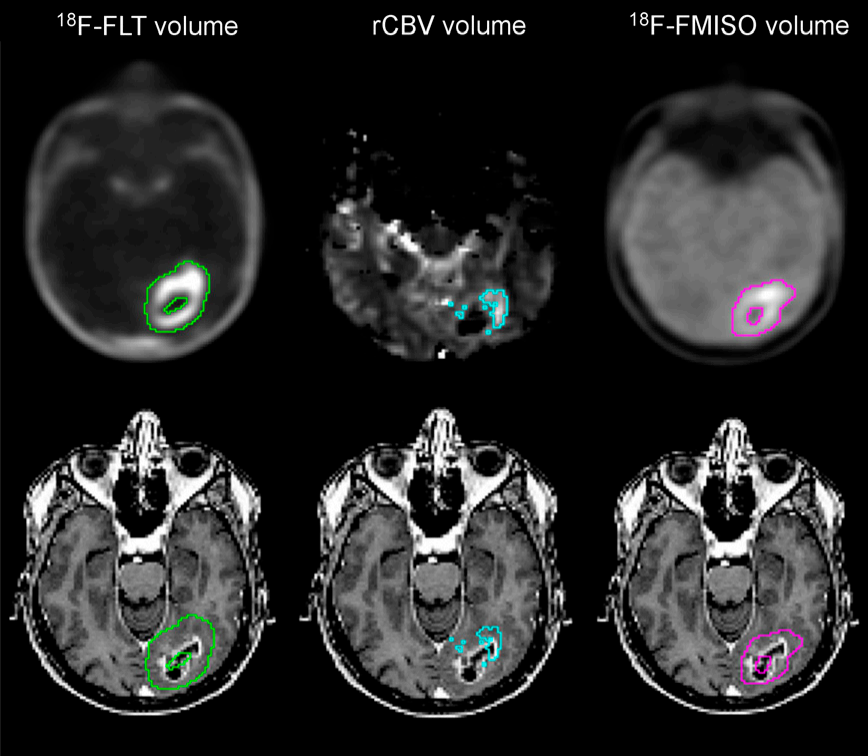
Example of proliferative volume in green, hypervascularized volume in blue, and hypoxic volume in pink on segmented (top) and overlaid (bottom) 3D T1w-Gd MR images.
